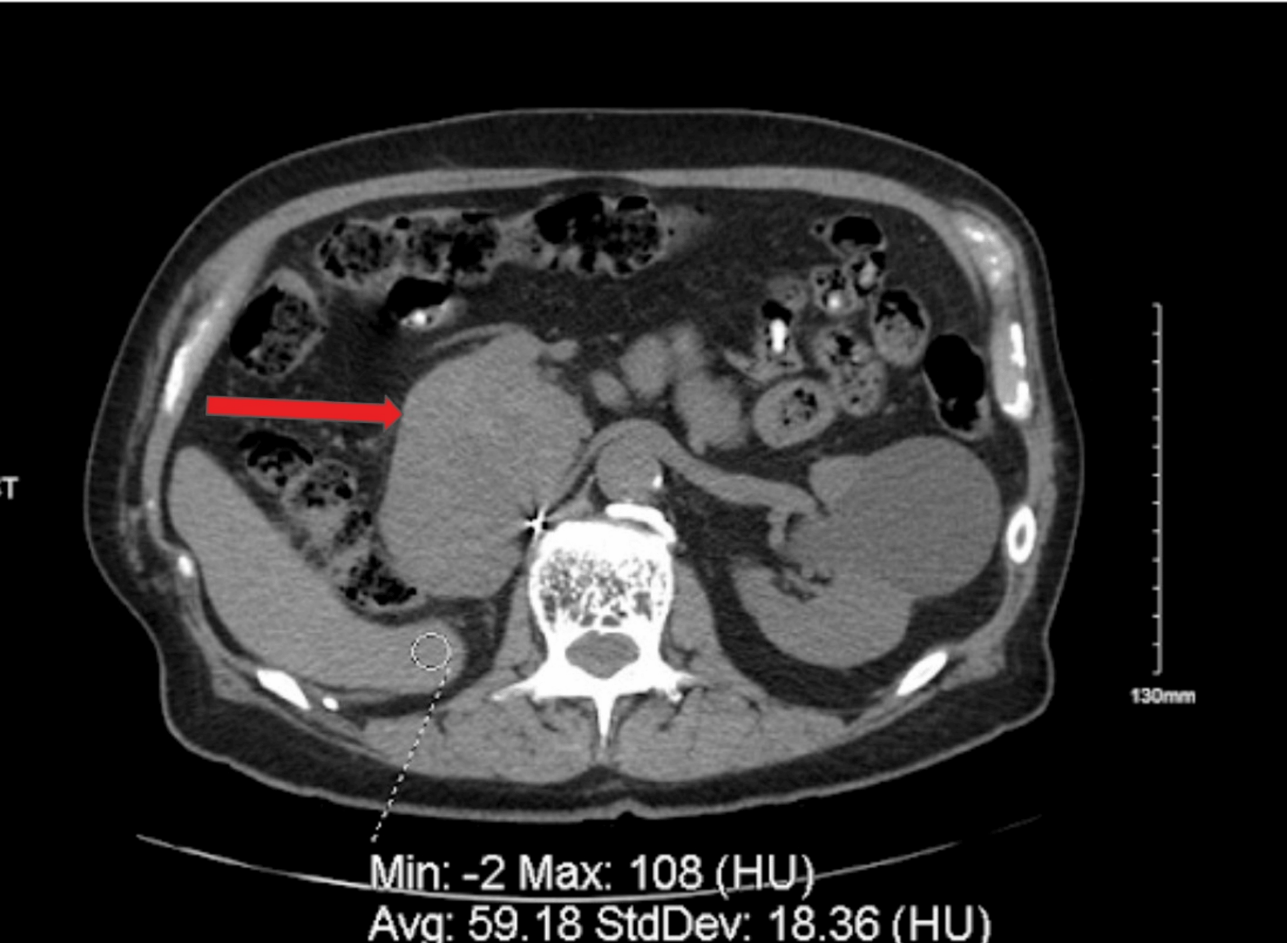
Axial abdominal computed tomography image The arrow indicates the pancreatic mass.
